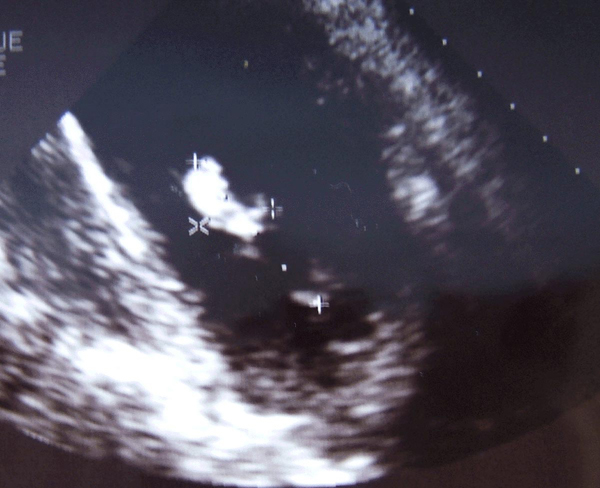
Transthoracic echocardiography showing large vegetations attached to the prosthetic mitral valve.
